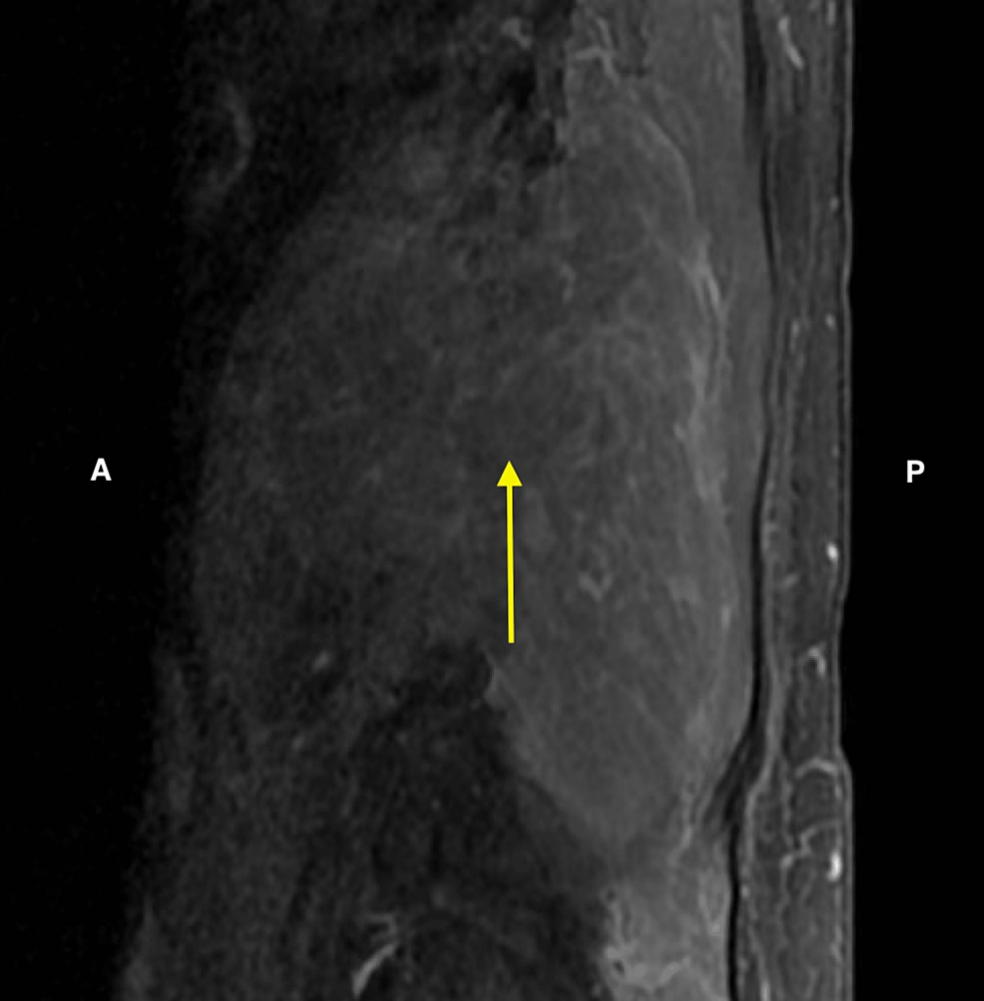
The interconnected anterior and posterior soft tissue components shown in the T1-weighted sagittal view with contrast A = Anterior; P = Posterior
